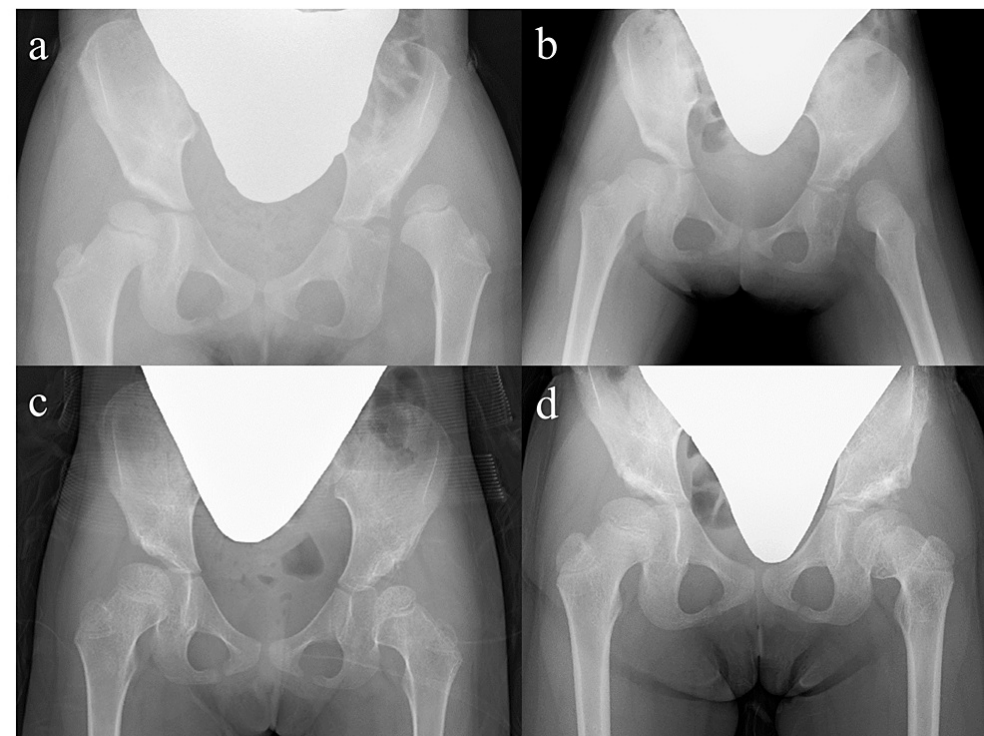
Supine anteroposterior hip radiographs of Case 2. (a) An initial hip radiograph obtained at five years and zero months of age showing a left-sided developmental dysplasia of the hip with Yamamuro’s “distance a” of −7 mm. (b) A follow-up hip radiograph obtained after 49 days of horizontal skin traction showing a sufficient “distance a” of 0 mm. (c) A follow-up hip radiograph obtained after treatment with a flexion-abduction brace showing the residual acetabular dysplasia of the left hip. (d) The latest hip radiograph obtained three years after Salter innominate osteotomy (SIO) demonstrating an apparent coxa vara with shortening of the femoral neck. The femoral head is slightly enlarged but seems to preserve sphericity. She underwent SIO for the acetabular dysplasia of the right hip at eight years and four months of age.
